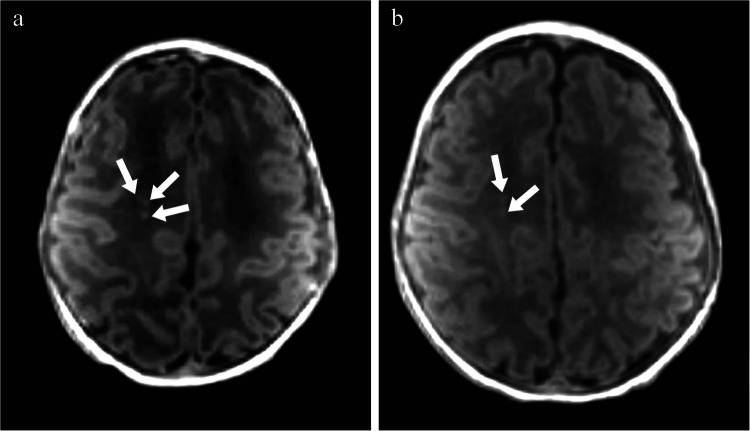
Axial T1 magnetic resonance images without contrast of a girl born at 35 + 6 weeks. a The image obtained on day 7 (36 + 6 weeks) shows more than three areas of hyperintensity, some > 2 mm, indicating moderate white matter injury (*arrows*). b The image obtained on day 31 (40 + 2 weeks) shows decreased intensity and size of the largest two lesions and apparent resolution of the third (*arrows*)
